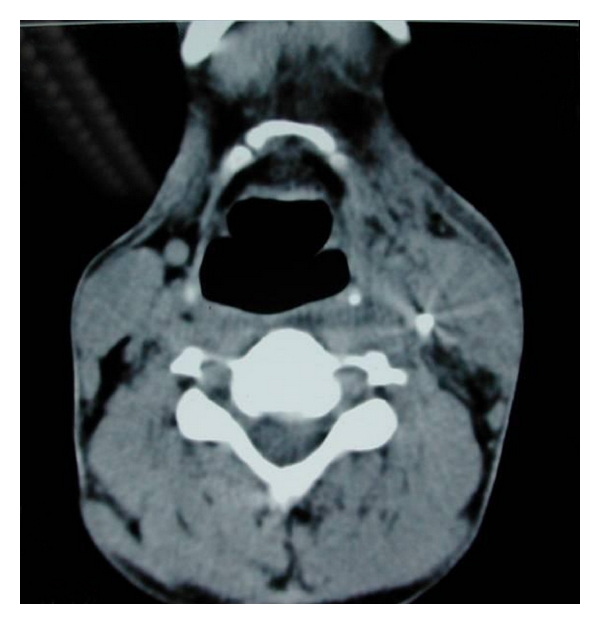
An axial CT scan showing the foreign body deep to the sternomastoid muscle lying in the parapharyngeal space.
